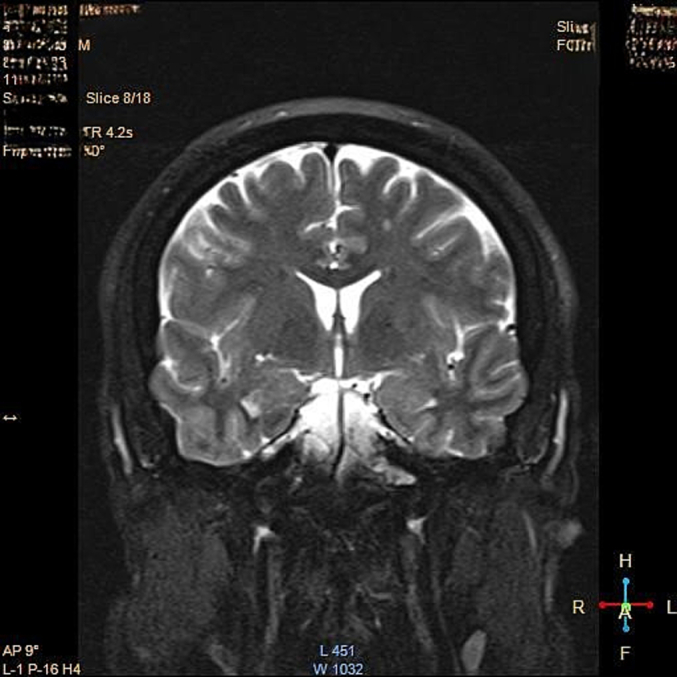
Post-operative coronal MRI image showing resolution of the lesion in the hypothalamic-pituitary region after surgical intervention, with no visible residual mass or significant postoperative complications.
